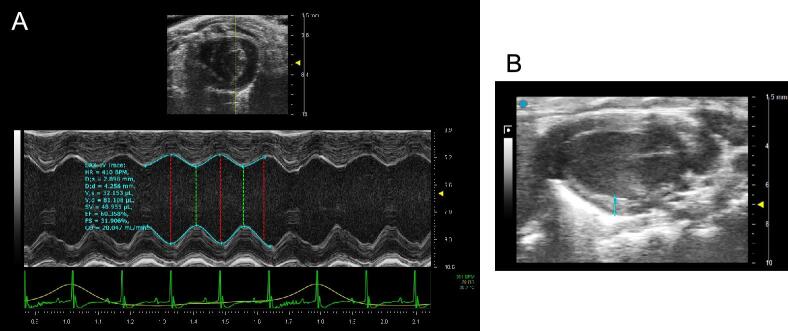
Settings on the Vevo 3100 ultrasound system. (A) LV parameters were calculated from the parasternal short-axis M-mode view, using manual tracing at the level of two papillary muscles. (B) LVPW thickness during diastole was measured on B-mode long-axis view by tracing the LVPW at the midpoint of the heart.
